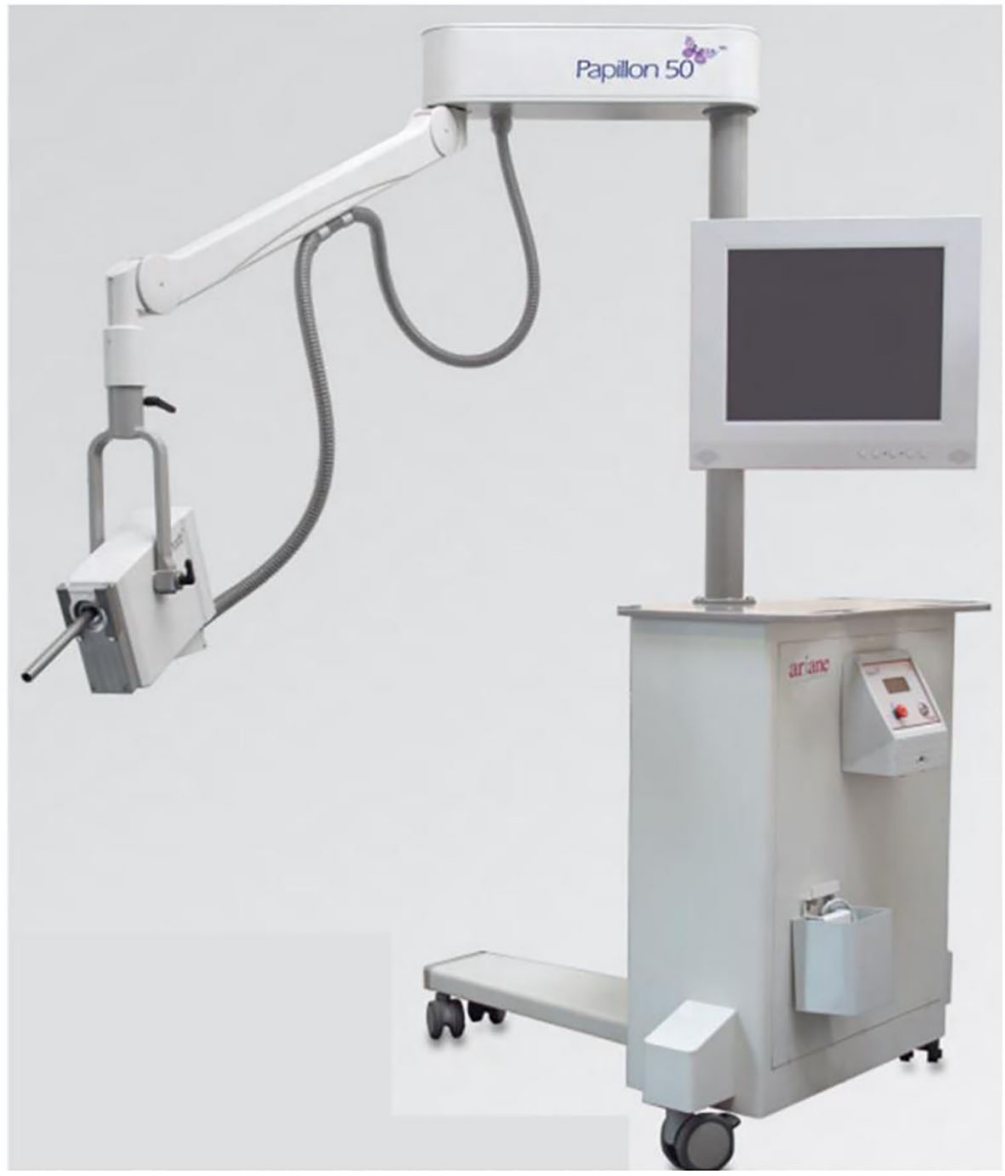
The Papillon 50™ contact X-ray brachytherapy system, equipped with a low­energy X-ray tube and interchangeable applicators of various diameters. The compact design allows outpatient delivery of high surface doses for rectal tumors.
